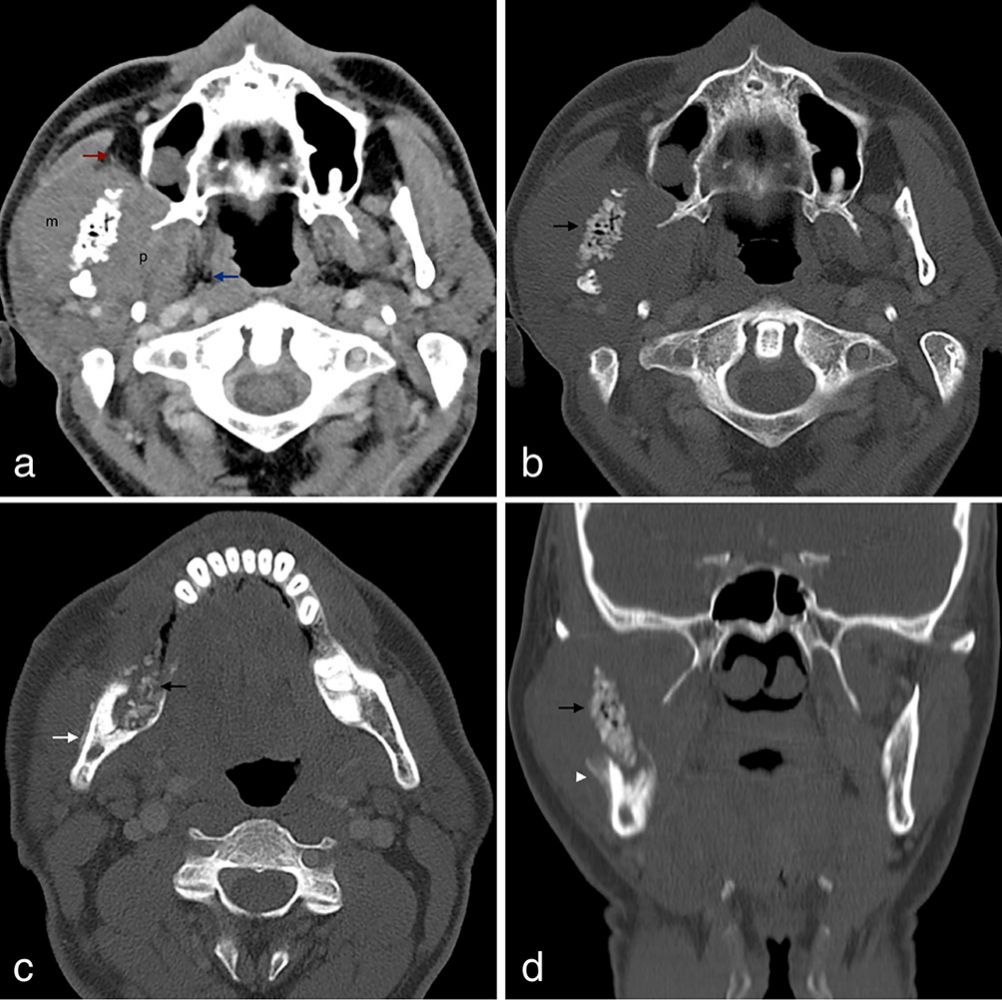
(a) Axial enhanced CT image in soft tissue window settings reveals a large right mandibular tumour consisting of a soft tissue component surrounding a markedly irregular and sclerotic mandibular ramus with some gas bubbles within the medullary cavity. The soft tissue component invades the masseter muscle (m) laterally and the pterygoid muscles (p) medially. The ipsilateral parapharyngeal space (blue arrow) and the Bichat’s fat pad (red arrow) are medially and anteriorly displaced, respectively. (b–d) Axial and coronal CT images in bone window settings better depict the heterogeneous bone involvement with a more irregular and sclerotic pattern in the mandibular ramus occupying the central portion of the tumour (black arrows) and a more expansive, remodelling pattern in the angle and posterior body with a ground-glass pattern of bone-forming matrix. Different patterns of periosteal reaction are depicted lengthwise in the mandibular ramus, including a thick regular deposition resembling an “onion skin” (white arrow) and a ruptured Codman triangle (white arrowhead).
